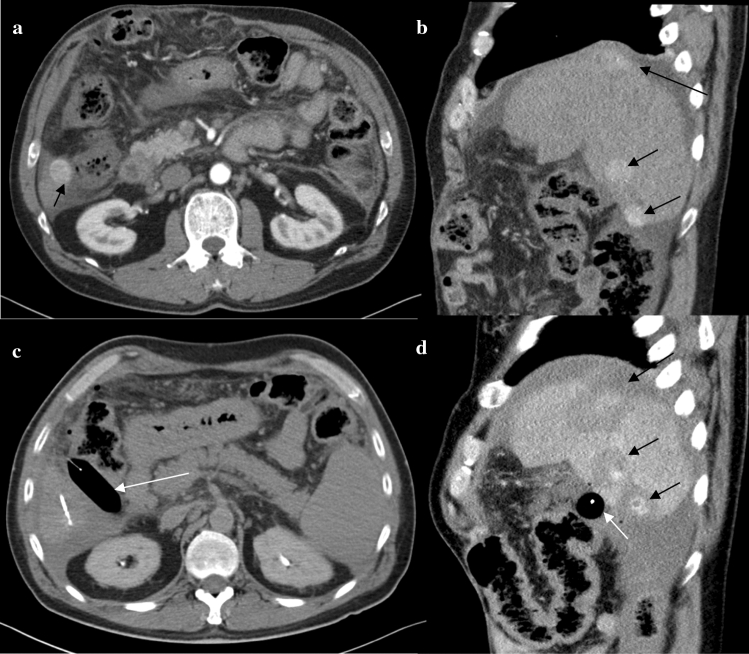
a, b Preinterventional CT-scan of a 60-year old male patient with liver cirrhosis and multifocal HCC (black arrows). Short distance between HCC tumours in S6 and the right colonic flexure. c MW ablation of the lesion in S6 following interposition of an angioplasty balloon (white arrow) between the liver and the colon. d Postinterventional CT-scan showing the balloon positioned between the ablation zone and the colon
